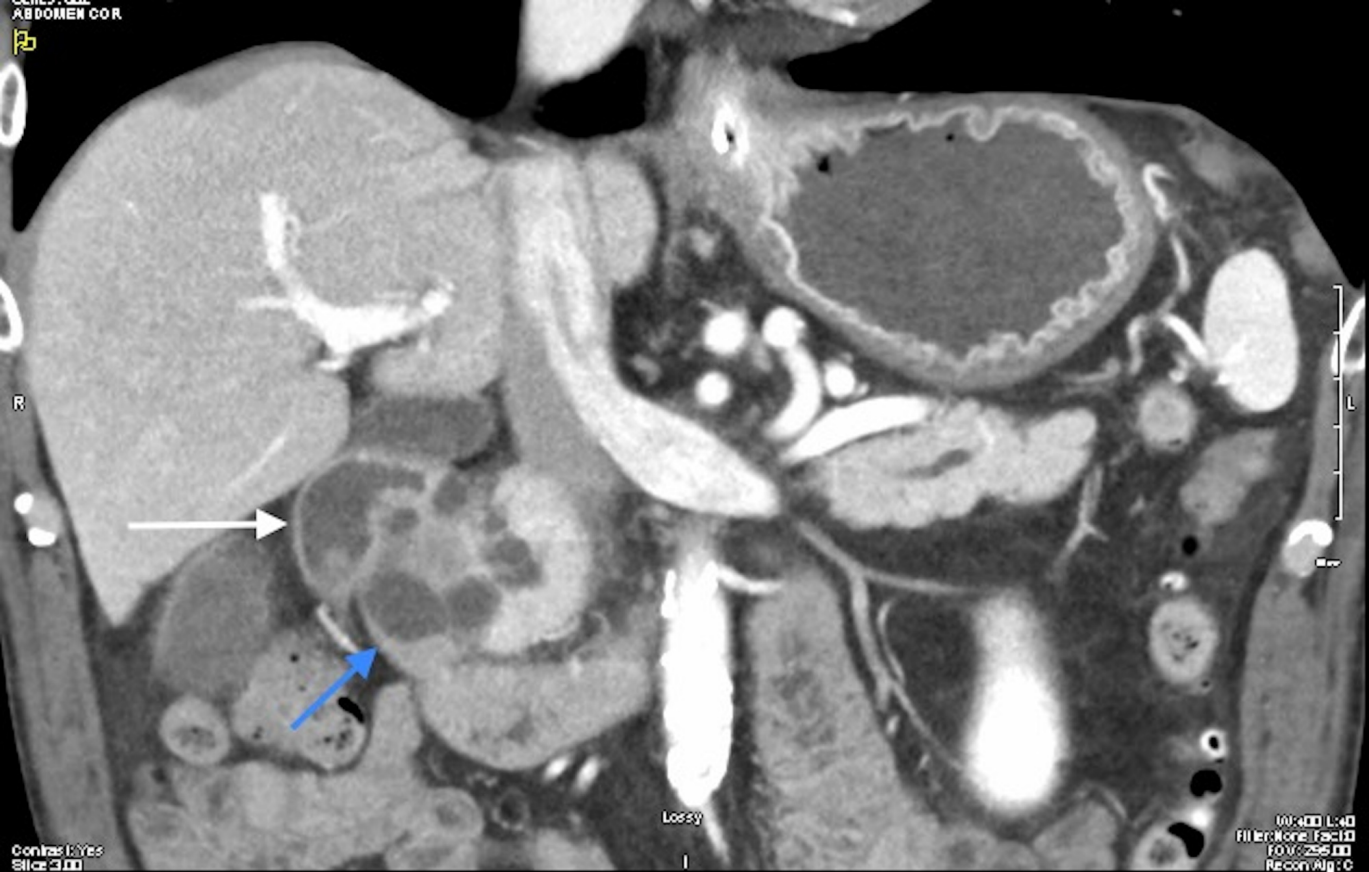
Coronal view abdominal CT scan with IV contrast in arterial phase White arrow: showing second part of duodenum; Blue arrow: cystic mass compressing duodenum.
